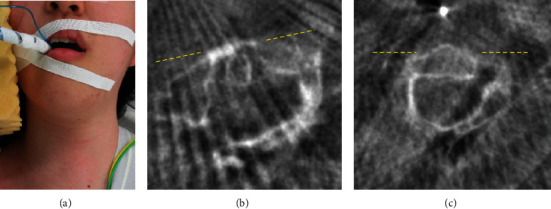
Patient's neck position (a) and CT after the first reduction (b, c). (b) Axial view of C1 and (c) axial view of C2. The C1-C2 rotatory angle decreased to 15 degrees.
